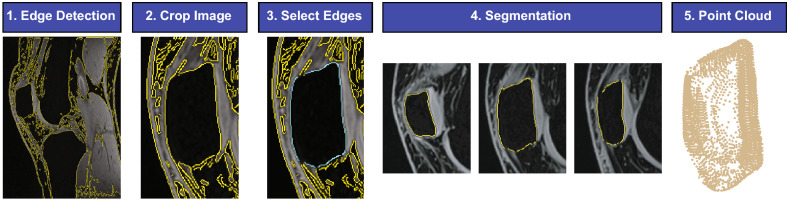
Semi-automatic segmentation steps for isolating bone from either MR or CT images. (1) A Canny edge detection filter is used to identify all edges in each image. The edges are overlaid in yellow on each image. (2) The image volume is cropped around the bone of interest (patella). (3) On a single starting image slice, the user selects the edges of the patella previously identified by the Canny filter. The selected edges are highlighted in cyan. (4) This starting slice is then used to determine the edges nearest these points in adjacent slices in the image volume. The user can remove stray edge points in any slice before proceeding. (5) The identified bone edges are converted into a three-dimensional point cloud.
